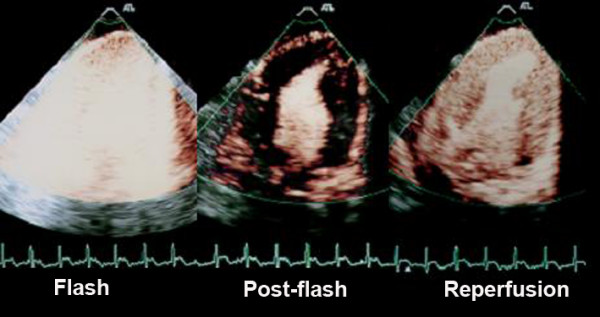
Example of a baseline representative study showing real-time myocardial contrast echocardiography with flash imaging used to cause myocardial microbubble destruction on the left, followed by absence of myocardial contrast in post-flash imaging (middle) and myocardial replenishment on the right, where complete and homogeneous myocardial perfusion can be observed. Sequences of myocardial replenishement were acquired at baseline, during coronary occlusion, after 30 minutes of reperfusion and with adenosine infusion.
